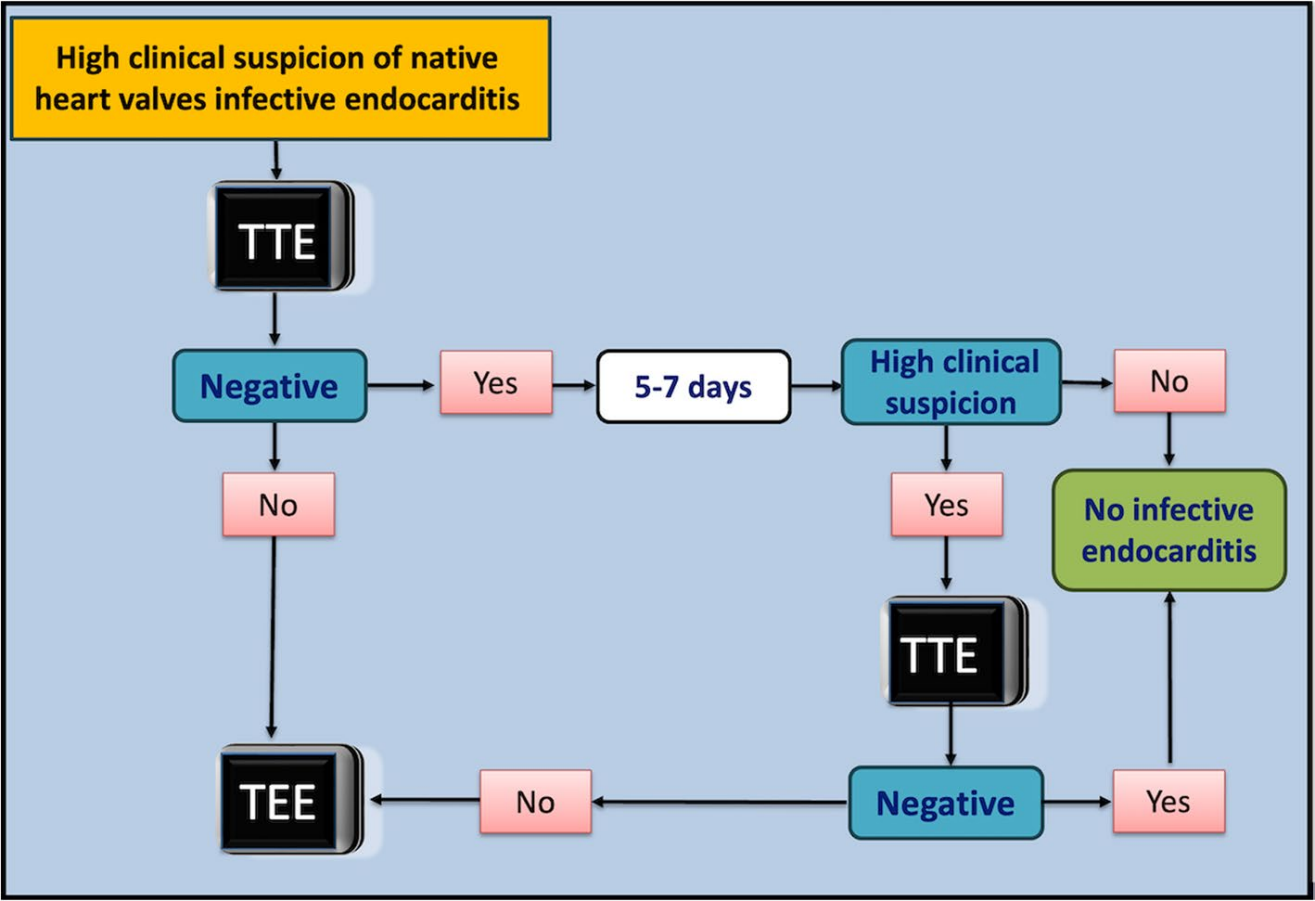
Diagnostic approach for patients with high clinical suspicion of native valve infective endocarditis. TEE, transoesophageal echocardiography; TTE, transthoracic echocardiography
